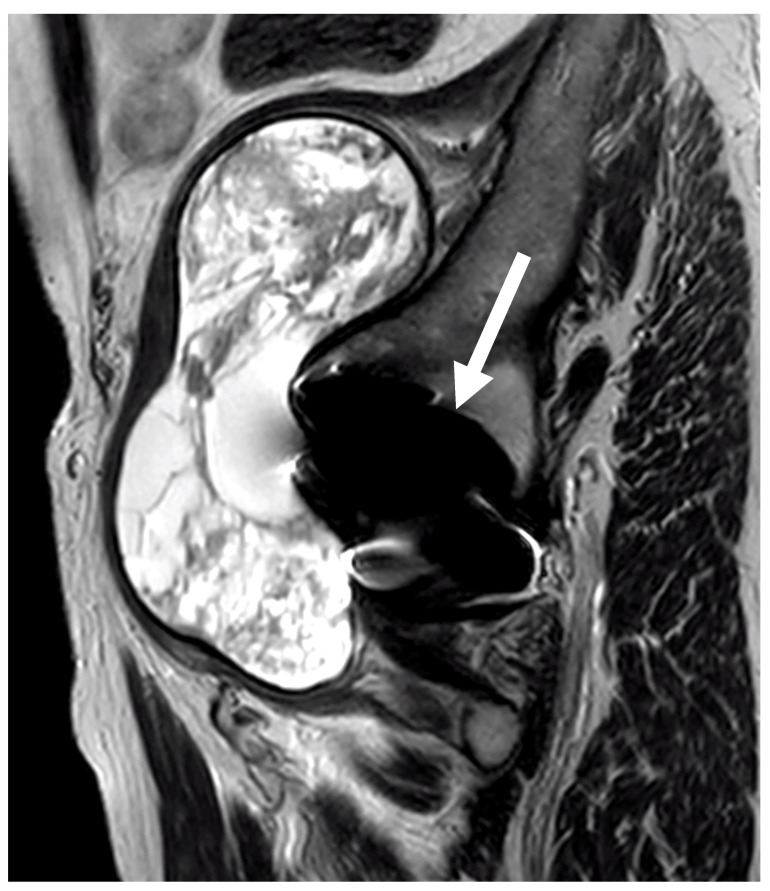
MRI image of a type III ALTR (white arrow) using MARS on the right hip.
